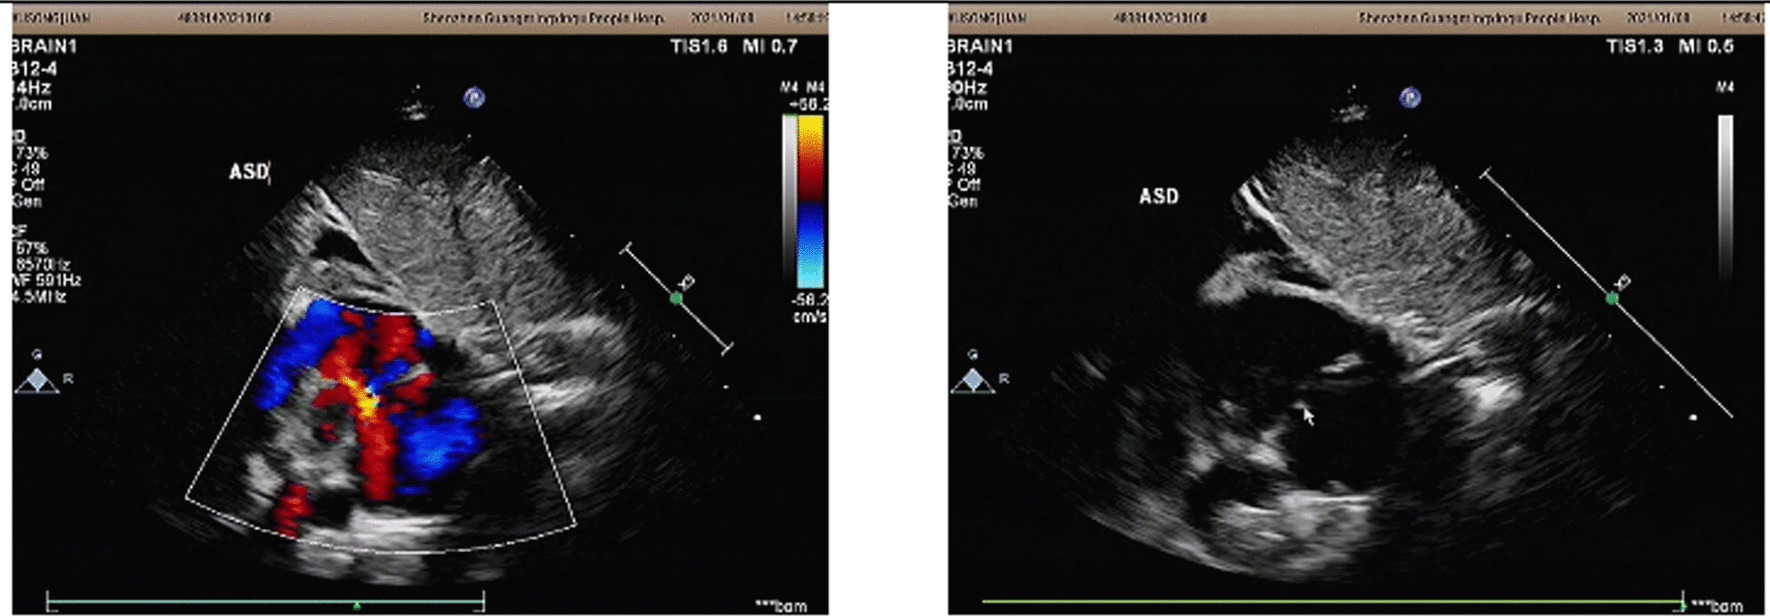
Image of Color Doppler echocardiography
